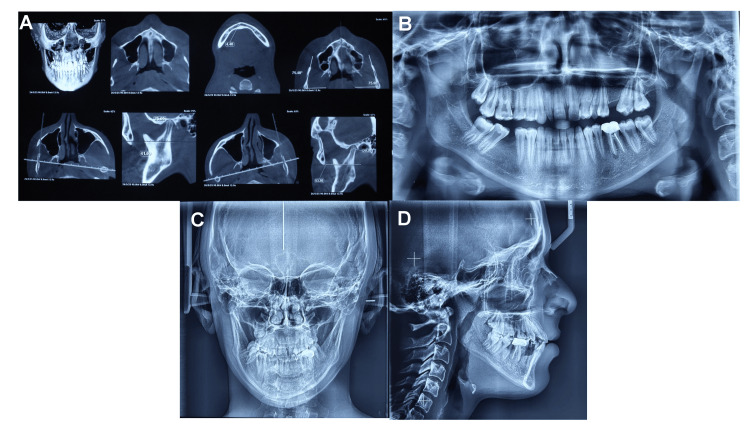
Pre-treatment radiographs A) CBCT B) Panoramic view C) PA cephalogram D) Lateral cephalogram
